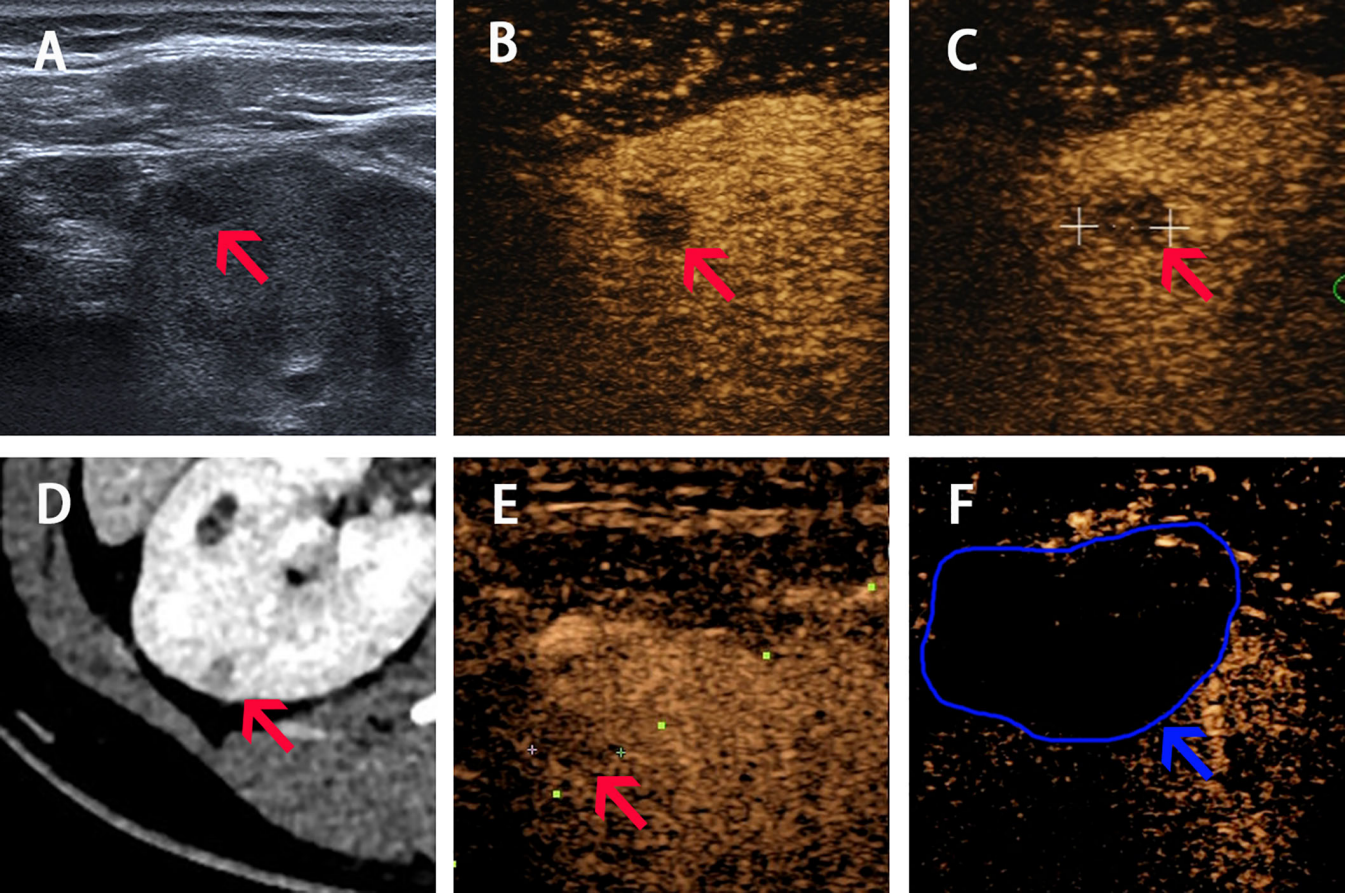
Imaging of Patient 2 before, during and after ablation procedure. (A–C) Ultrasound image acquired in prone position before ablation shows a slight hypoechoic nodule of 6-mm diameter with hypo-enhancement in both cortical phase (24 s) and medulla phase (70 s) (pointed by red arrow). (D) Arterial phase of CT before procedure shows a 6-mm diameter nodule located in the right kidney (pointed by red arrow). (E) The real-time CEUS guided the locating of nodule during ablation (red arrow shows the lesion and the green dotted line shows the puncture path). (F) CEUS showed non-enhancement during both cortical and medulla phases postprocedure (pointed by blue arrow and curve).
